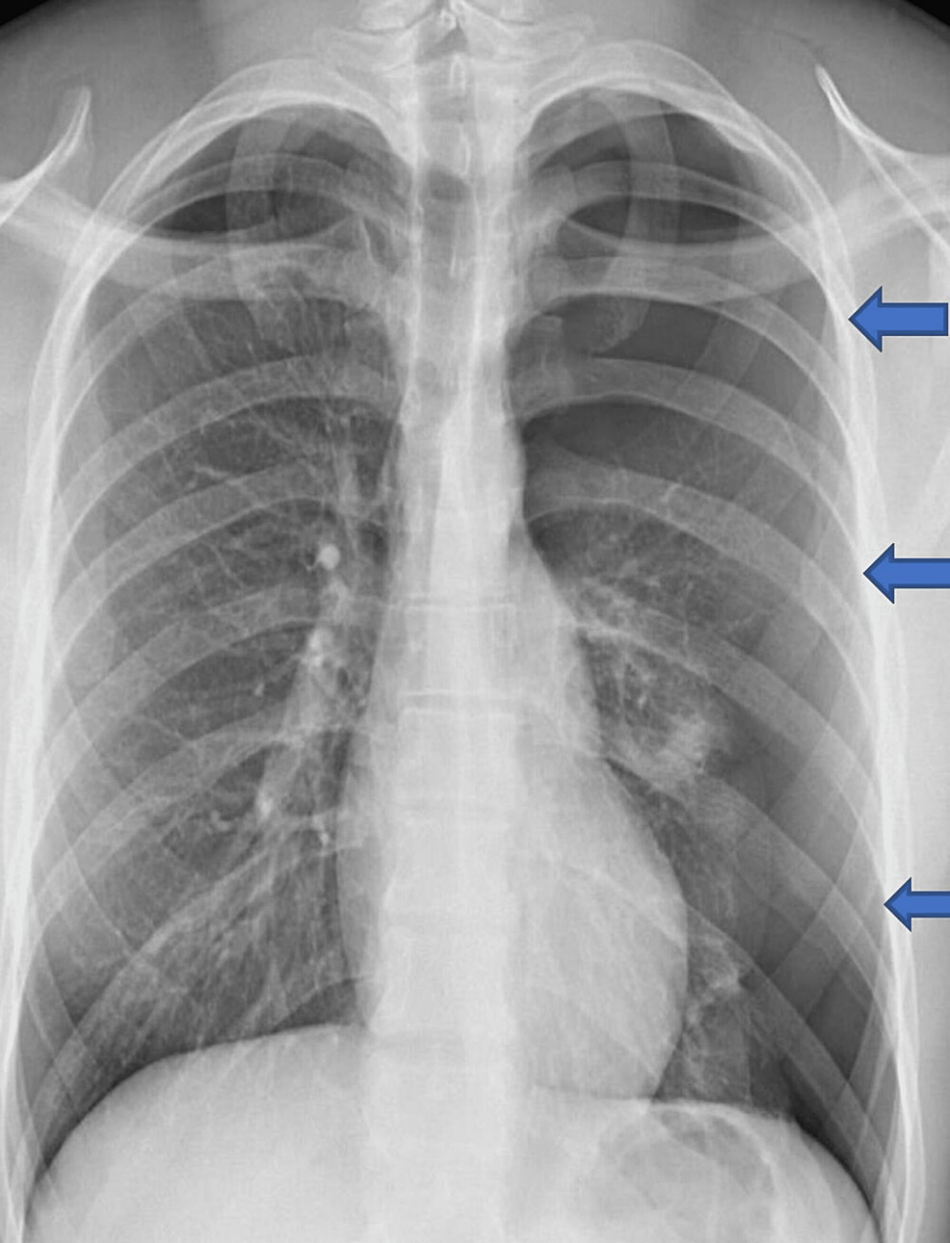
Chest radiography showing left-sided pneumothorax as shown by the arrows.
